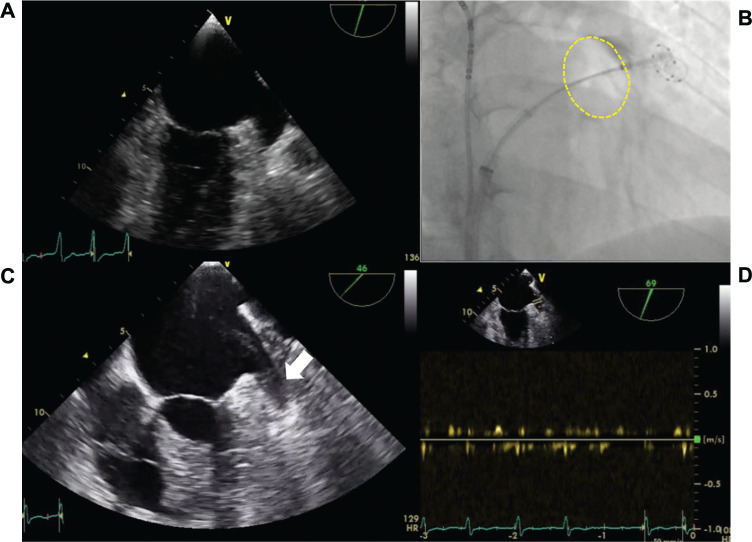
A: Preprocedural transesophageal echocardiography (TEE) revealed the absence of thrombus or spontaneous echocontrast (SEC) within the left atrial appendage (LAA). B: Successful occlusion of the LAA using a third-generation cryoballoon (yellow dashed contour)—right anterior oblique 30° view. C: Postprocedural TEE at the third month indicated grade 2 SEC (white arrow), but there is no evidence of thrombus formation within the LAA. D: LAA velocity was measured at 0.24 m/s.
